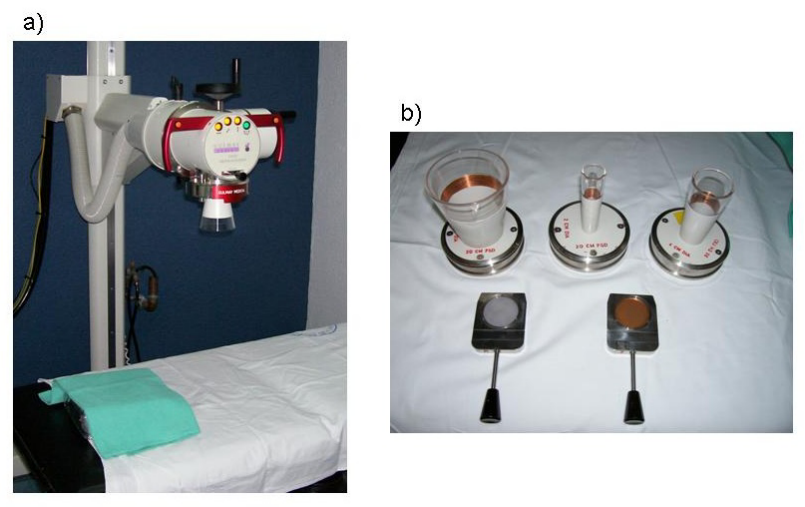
a) Orthovoltage X-ray unit (Gulmay D3225); b) filters and applicator cones.
